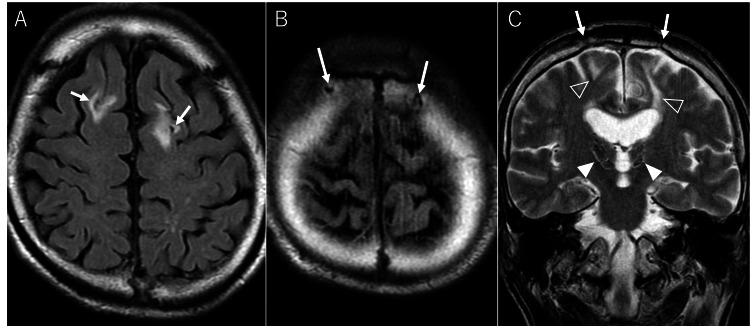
Trajectory to the thalami. FLAIR images reveal symmetrical hyperintense areas in bilateral frontal lobes (A), suggesting encephalomalacia. Above these areas, defects in bilateral parietal bones (B) like post-puncture changes were observed. On the T2WI coronal image (C), the parietal bone (arrow), frontal lobe (open arrowheads), thalamic, and subthalamic nucleus lesions (arrowheads) align linearly through the lateral ventricles. FLAIR: fluid-attenuated inversion recovery.
